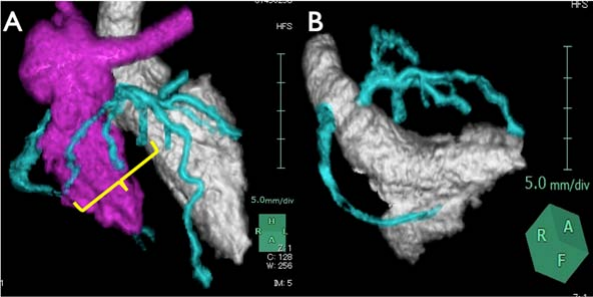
a, b Three-dimensional CT coronary angiography showed the conus branch salvaged (*square bracket*) and enough space between the left coronary artery and the left pulmonary artery. The elongated left main trunk was very smooth without stenosis
